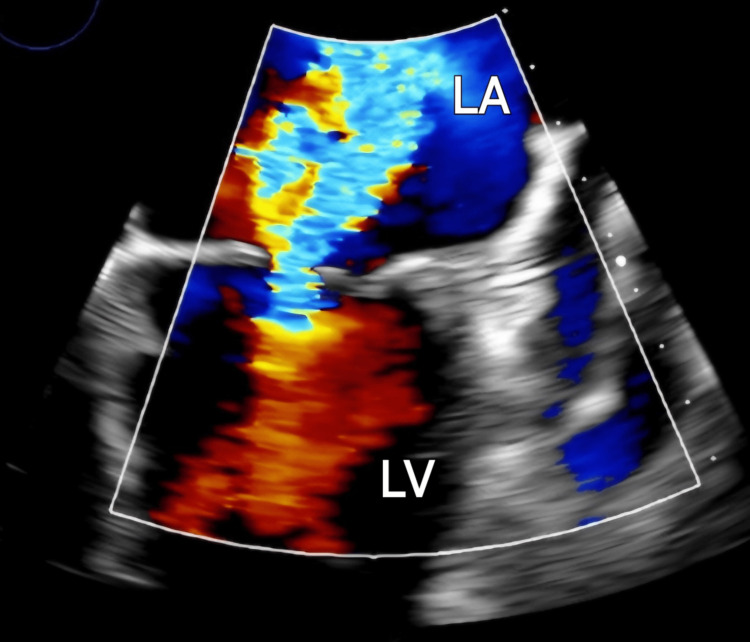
Transthoracic echocardiogram revealing severe mitral regurgitation and moderate to severe mitral stenosis Thickened and calcified mitral leaflets with diastolic doming (hockey-stick deformity) and restriction of the posterior leaflet, with findings consistent with rheumatic heart disease. In addition, a central leaflet malcoaptation gap is present, resulting in significant regurgitation. Doppler evaluation demonstrates severe regurgitation with multiple centrally directed jets. The PISA radius is 1.28 cm, and the peak mitral regurgitation velocity is 454 cm/s. The effective regurgitant orifice area is 0.75 cm², and the regurgitant volume is 83 mL. PISA: proximal isovelocity surface area; LV: left ventricle; LA: left atrium
